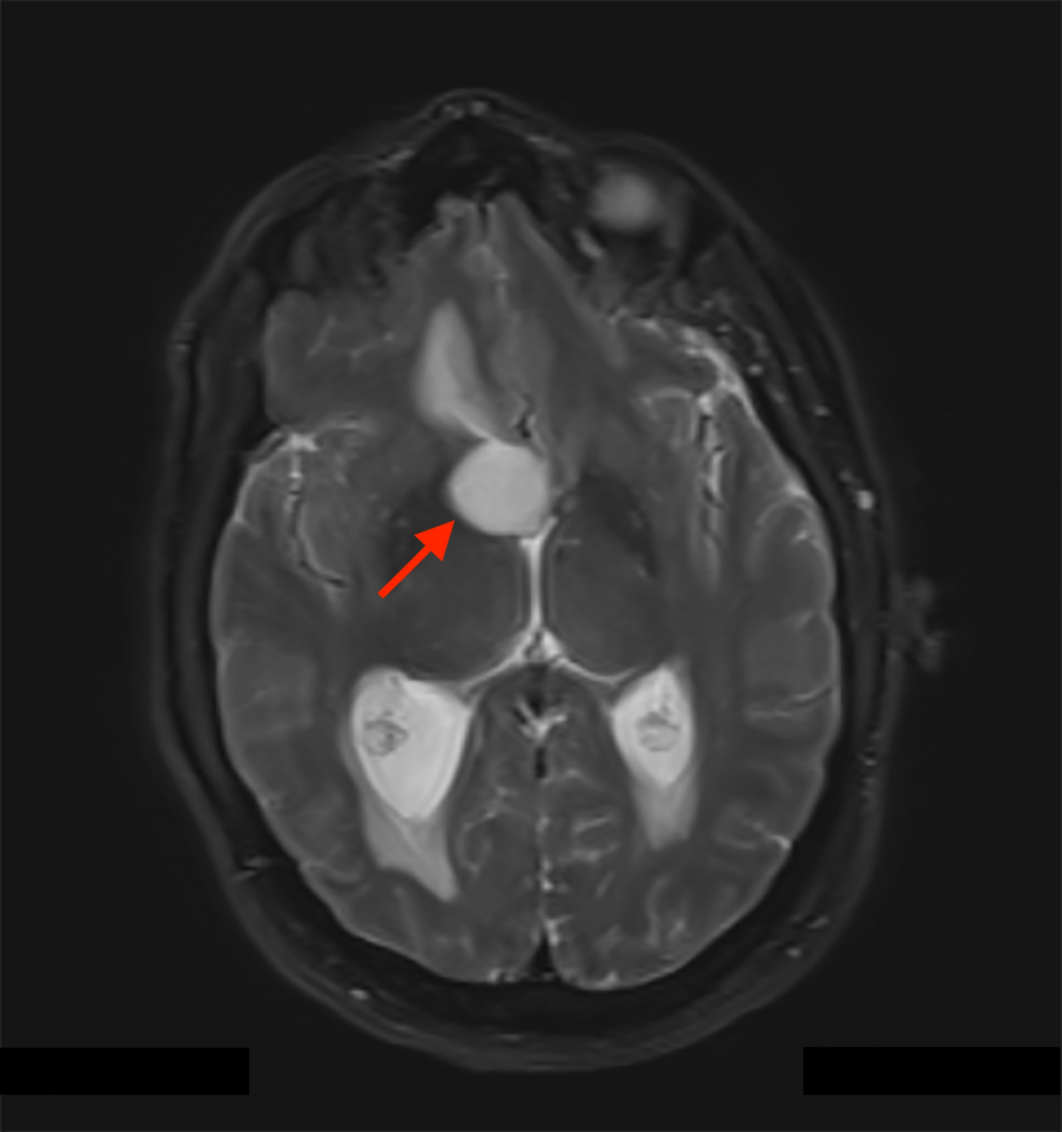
Axial T2 weighted MRI with the arrow indicating foramen of Monro. The lesion is a cystic-appearing intraventricular mass with associated obstructive hydrocephalus and no restricted diffusion.
